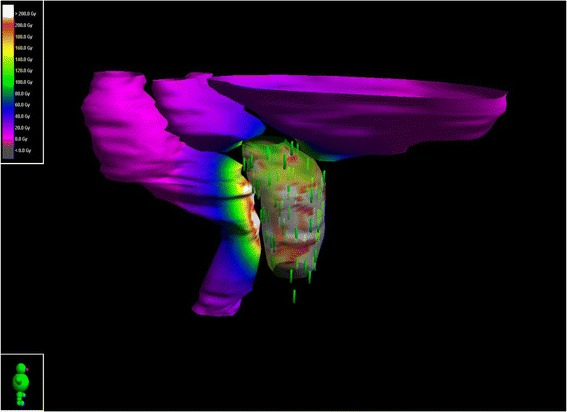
Typical dose distribution following ^125^I prostate brachytherapy. The rectum, prostate and bladder have been contoured on a CT scan 4 weeks after brachytherapy and reconstructed in 3D. Brachytherapy seeds within the prostate are highlighted green and a colourwash representing total dose has been applied to the surface of each organ
